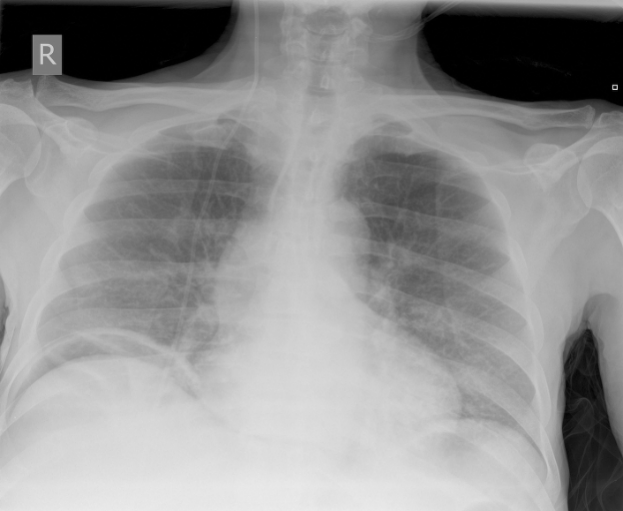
Chest X-ray showing free intraperitoneal air.
